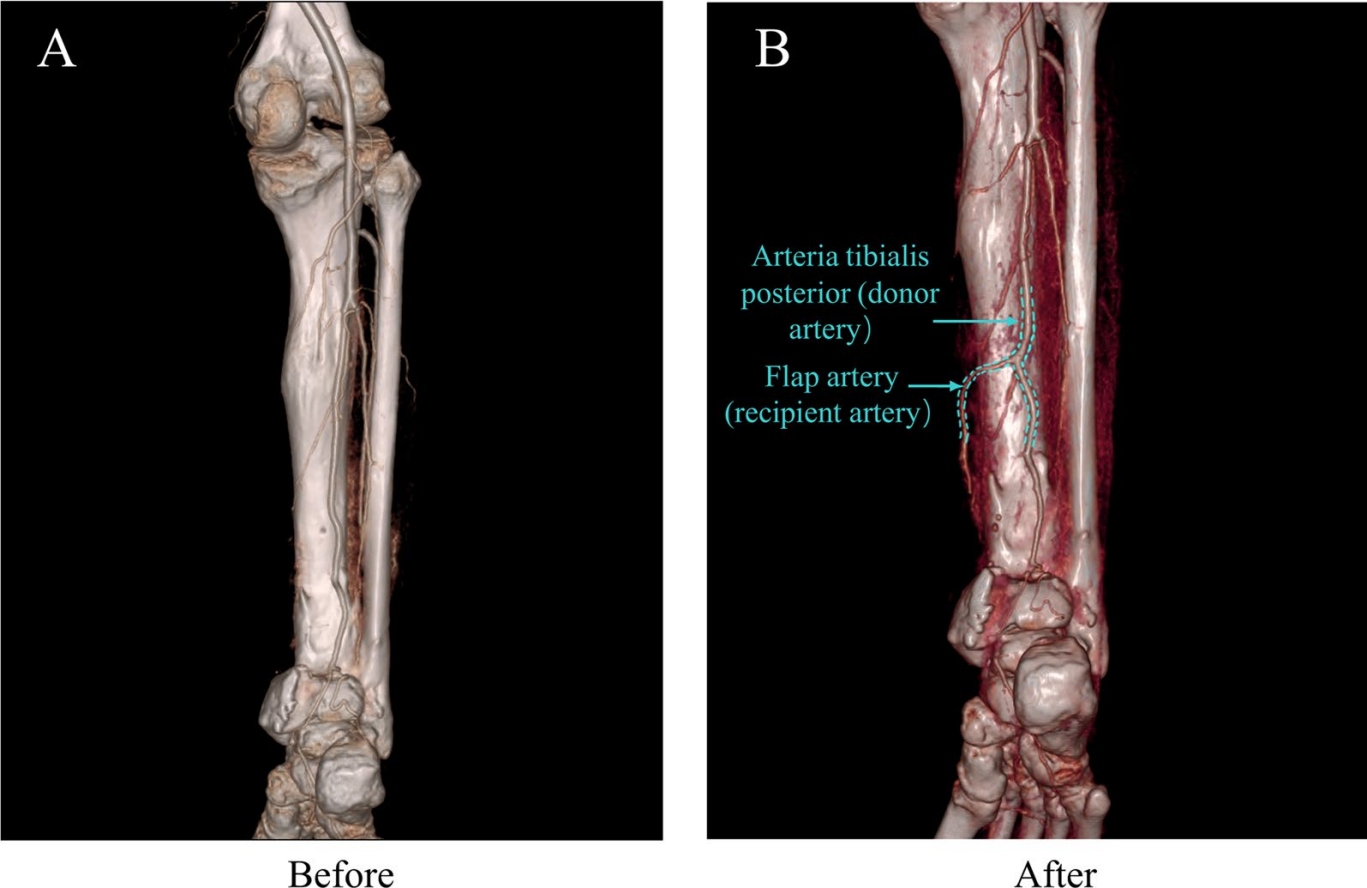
CTA examination of the donor and recipient arteries, where the images showed good patency in the anastomosis site post-surgery
